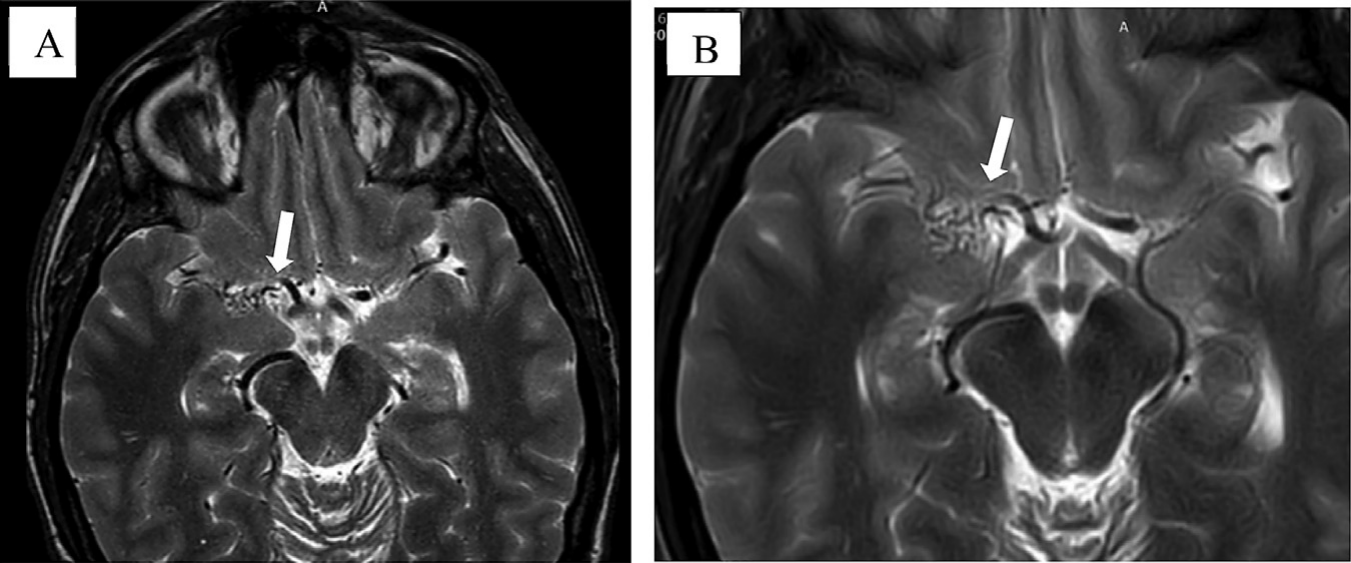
MRI Axial T-2 weighted images show no ischemic lesion. The flow-void signal of the right middle cerebral artery is sluggish, with diffuse net of small vessels denoting collaterals. (images A and B) (white arrows)
